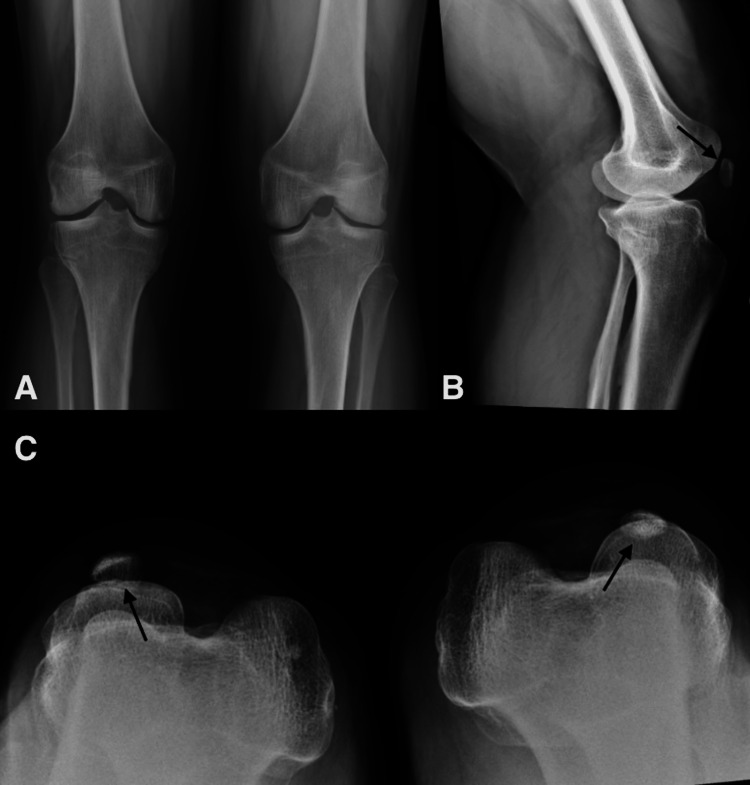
Anteroposterior (A), lateral (B), and sunrise (C) radiographs revealing bilateral tiny patellae (arrows)
